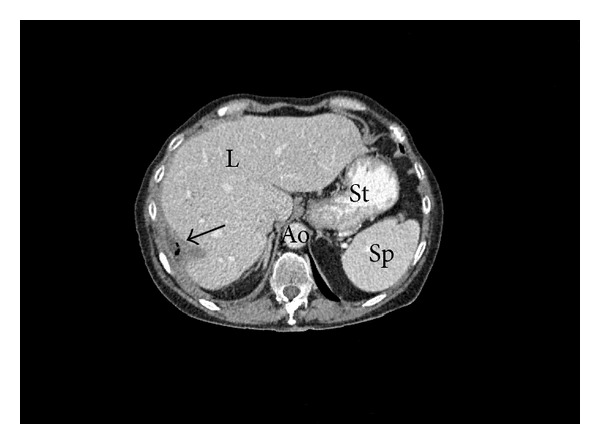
Computed tomography showing a complete healing of the subphrenic abscess (arrow); aorta (Ao); liver (L); spleen (Sp); stomach (St).
